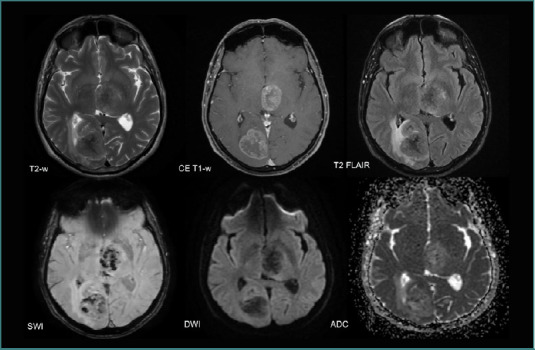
MRI brain acquisition of adenosquamous LC BMs
